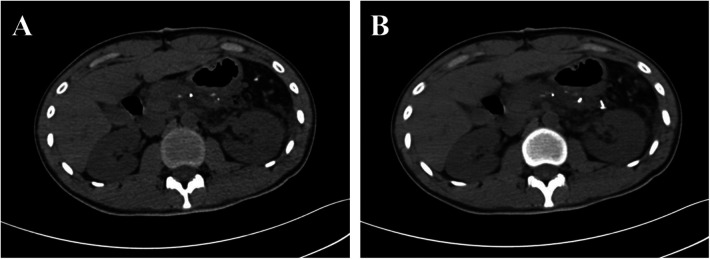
CT showed the patient was free from tumor recurrence after 2 years' following up. (A and B, Plain scan).
